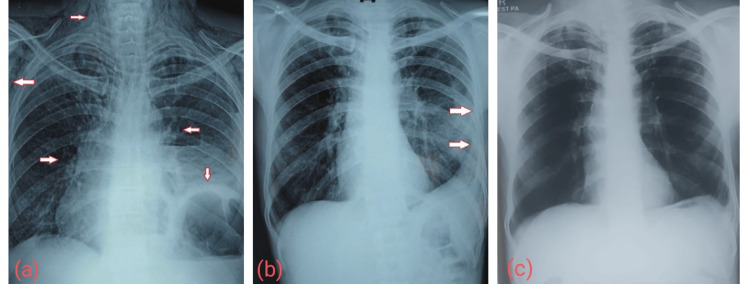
(a) Chest X-ray at presentation showing a raised left dome of the diaphragm and air-fluid level with pleural collection along with subcutaneous emphysema in the neck, chest, and axilla. (b) Postoperative chest X-ray showing left pleural thickening with no pleural collection. (c) Follow-up chest X-ray after six months showing good clearance of empyema.
